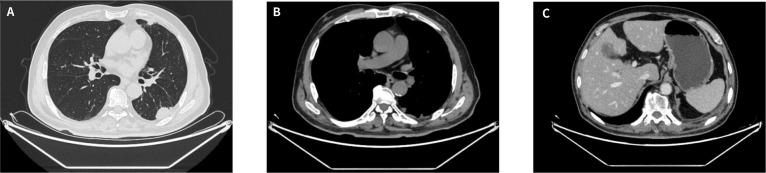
Follow-up chest CT obtained on December 31, 2025, during maintenance tislelizumab monotherapy. (A) Lung window image showing persistent reduction of the left lower-lobe pulmonary lesion compared with baseline. (B) Mediastinal window image showing no radiologic progression of residual pleural/subpleural lesions. (C) Mediastinal window image at the upper abdominal level showing no recurrent or increased left pleural effusion. Overall, compared with baseline, the findings are consistent with an ongoing partial response without evidence of local progression.
